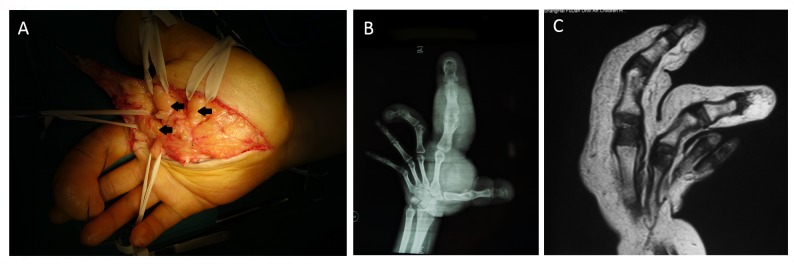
Surgical view and imaging of macrodactyly. (A) Enlargement in the digital nerve in type II macrodactyly (black arrows). (B, C) Adipose overgrowth and bone hypertrophy can be assessed by X-ray and magnetic resonance imaging; however, these techniques cannot accurately display nerve enlargement and functional damage.
